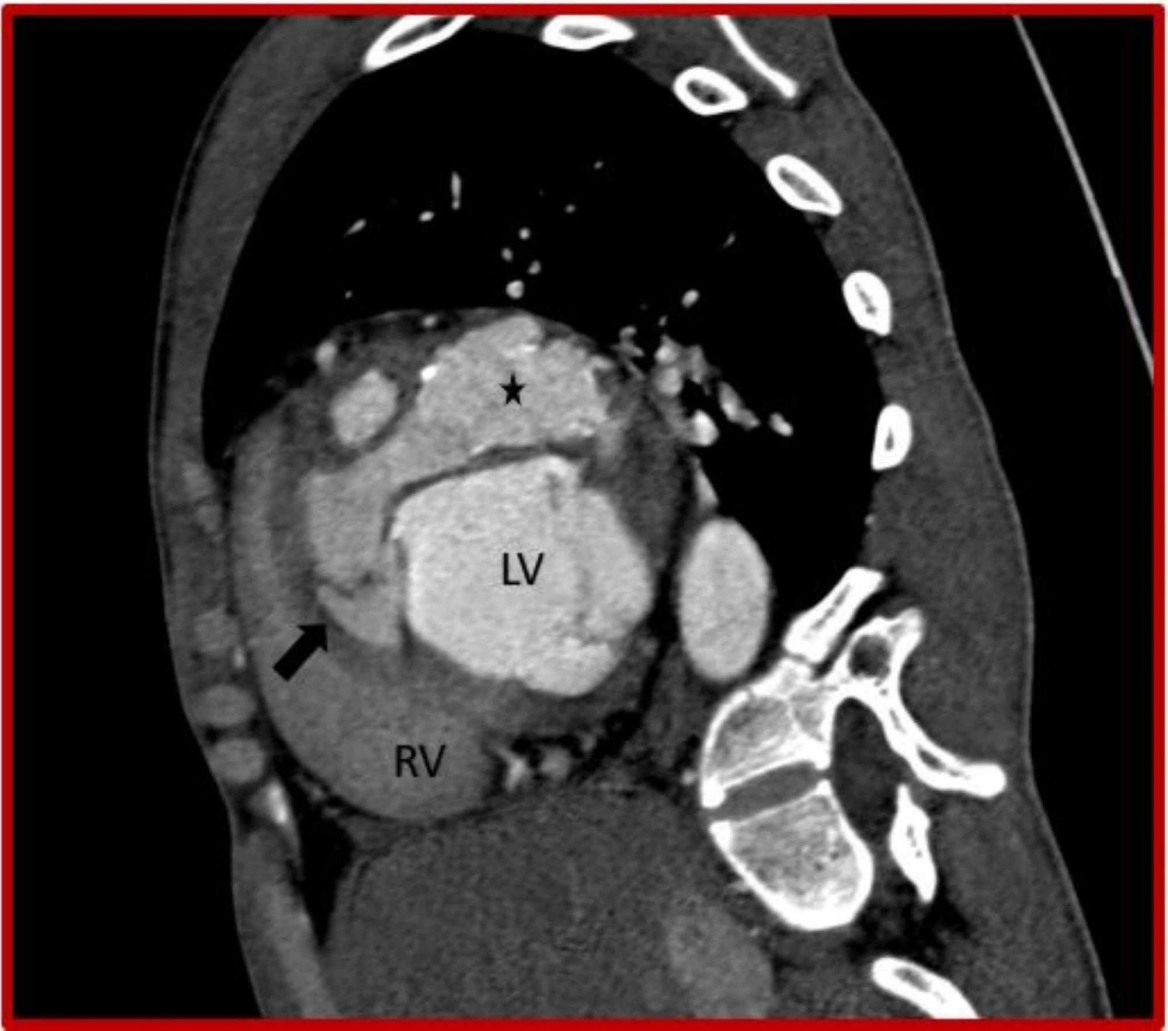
Cardiac CT (sagittal oblique view) demonstrates ruptured left sinus of Valsalva aneurysm (asterisk) dissecting into the interventricular septum (black arrow) from the basal to mid septal region. CT: Computed tomography; RV: Right ventricle; LV: Left ventricle.
